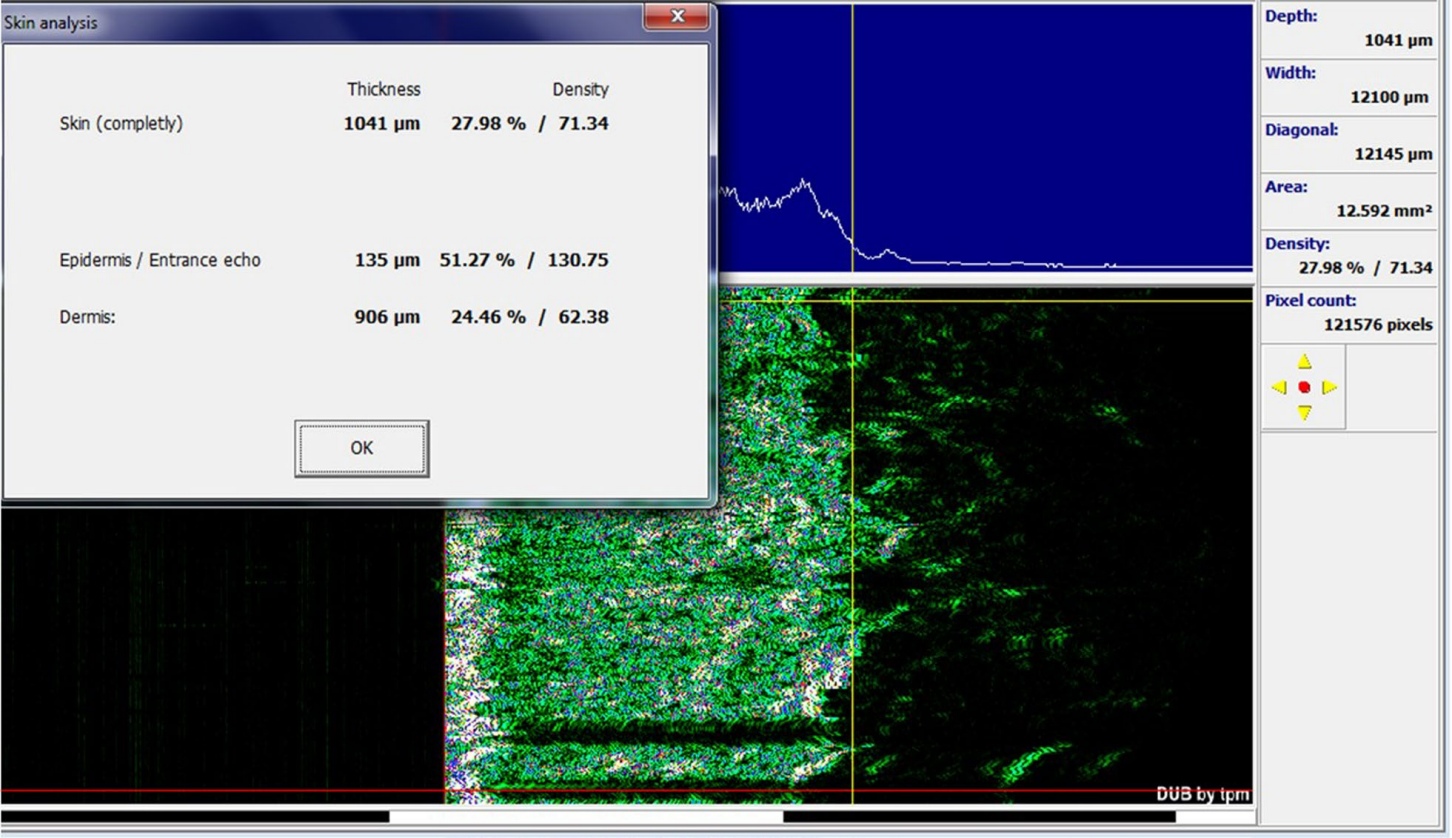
Sonographic findings after intervention with fractional CO_2_ laser
